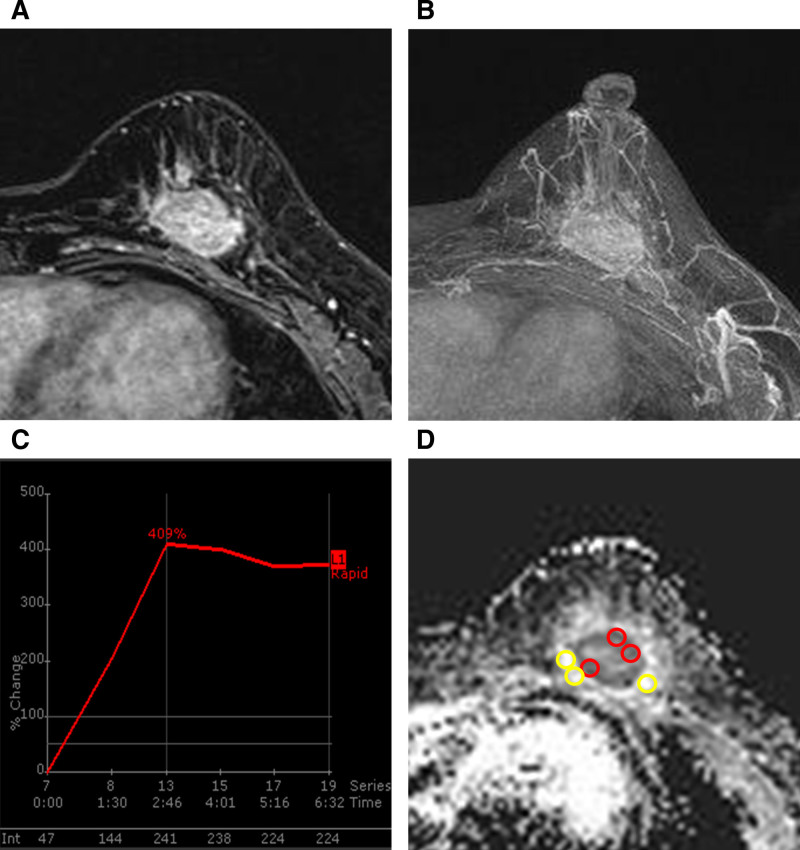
Breast magnetic resonance imaging findings of large cell neuroendocrine carcinoma. (A) The maximum intensity projection (MIP) image shows mildly increased ipsilateral whole-breast vascularity with the adjacent vessel sign. (B) Subtraction image from DCE-MRI revealed a mass in the 6 o’clock position of the left breast with rim internal enhancement. (C) The mass demonstrated a rapid initial enhancement followed by a washout pattern on the kinetic curve. (D) On the ADC map, the minimum mean value among the 3 ROIs measured within the tumor was 745 × 10^−6^ mm²/s, and the maximum value among the 3 ROIs measured in the brightest areas of the peritumoral lesion was 2075 × 10^−6^ mm²/s, resulting in a peritumoral-to-tumoral ratio of 2.79. ADC = apparent diffusion coefficient, DCE-MRI = dynamic contrast-enhanced magnetic resonance imaging, MIP = maximum intensity projection, ROI = region of interest.
